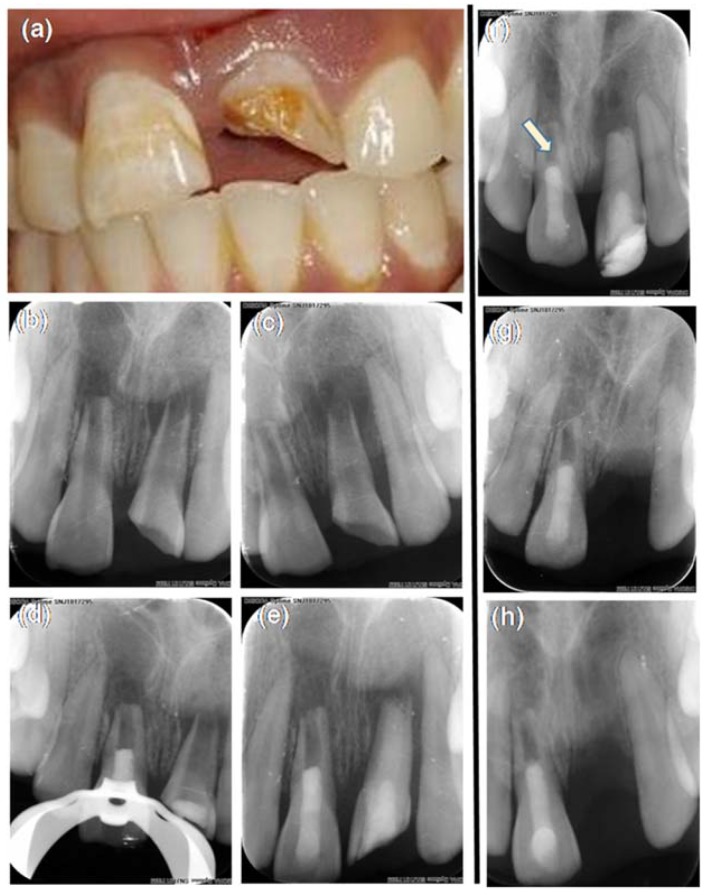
Intraoral periapical radiograph (IOPA) images of preoperative and a three-year follow up of REP in 11. (a) pre-operative photograph of maxillary central and lateral incisors; (b,c) pre-operative IOPA of teeth 11 and 21; (d) IOPA showing a coronal seal after placement of HAM in 11; (e) immediate postoperative IOPA of 11; (f) IOPA at a three-month follow up. The arrow is pointing to the calcific barrier above the Biodentine; (g) 19 month follow up IOPA; (h) three-year follow up IOPA.
